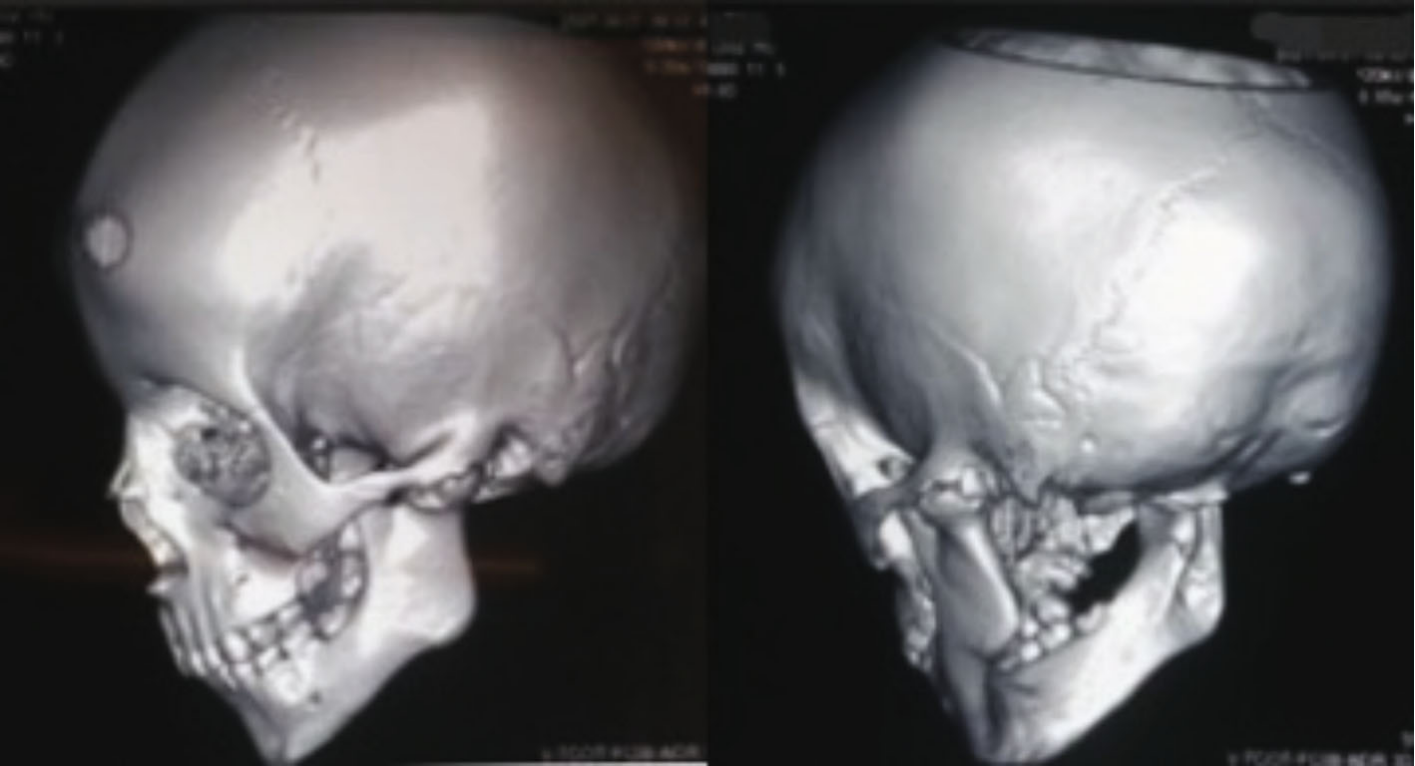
A 3-D reformatted CT scan of the patient showing left TMJ ankylosis and elongated left coronoid process.
